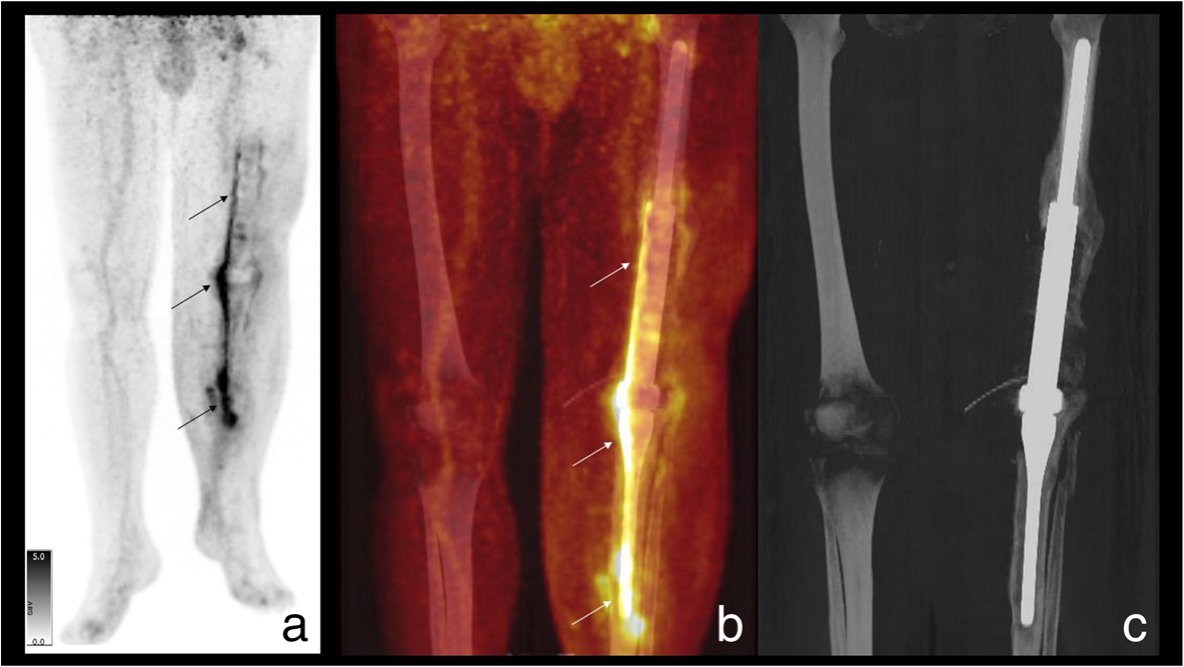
[18 F]FDG PET/CT: a Maximum intensity projection (MIP) of [18 F]FDG-PET; b MIP of [18 F]FDG PET/CT fusion and c MIP of CT (bony window): clearly increased glucose consumption along the medial side of the arthrodesis (arrows) and punctum maximum at the distal end with extension into the soft tissue as evidence of a prosthesis infection
